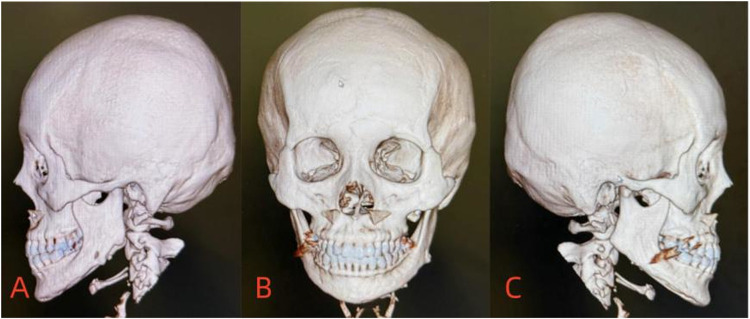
Preoperative CT-based craniofacial reconstruction showing the extent of midface concavity and any pre-existing implants. (A) Left oblique view. (B) Frontal view. (C) Right oblique view.
